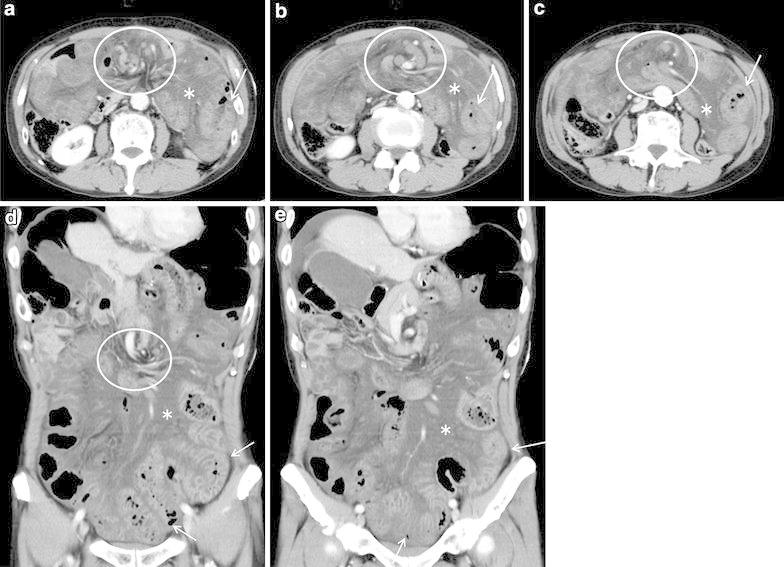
a–c Contrast-enhanced abdominal CT (90 s after injection of contrast agent) axial image of case 1; d, e contrast-enhanced abdominal CT (90 s after injection of contrast agent) coronal image of case 1. The dilated small intestine with a thickened bowel wall (*arrow*) with a high density area of mesenteric fat presenting with edematous changes (*asterisk*). Strangulation of the small intestine and mesenteric fat, and vascular structures presenting as the whirl sign (*circle*) can also be identified
